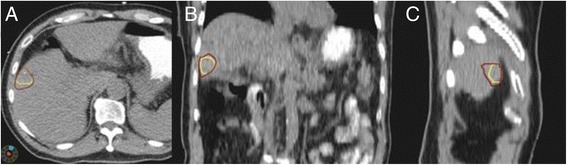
A comparison of IGTVs before and after DR in 4D CT images. A. axial view. B. coronal view. C. sagittal view. Yellow contours: IGTV before DR; red contours: IGTV after DR.
